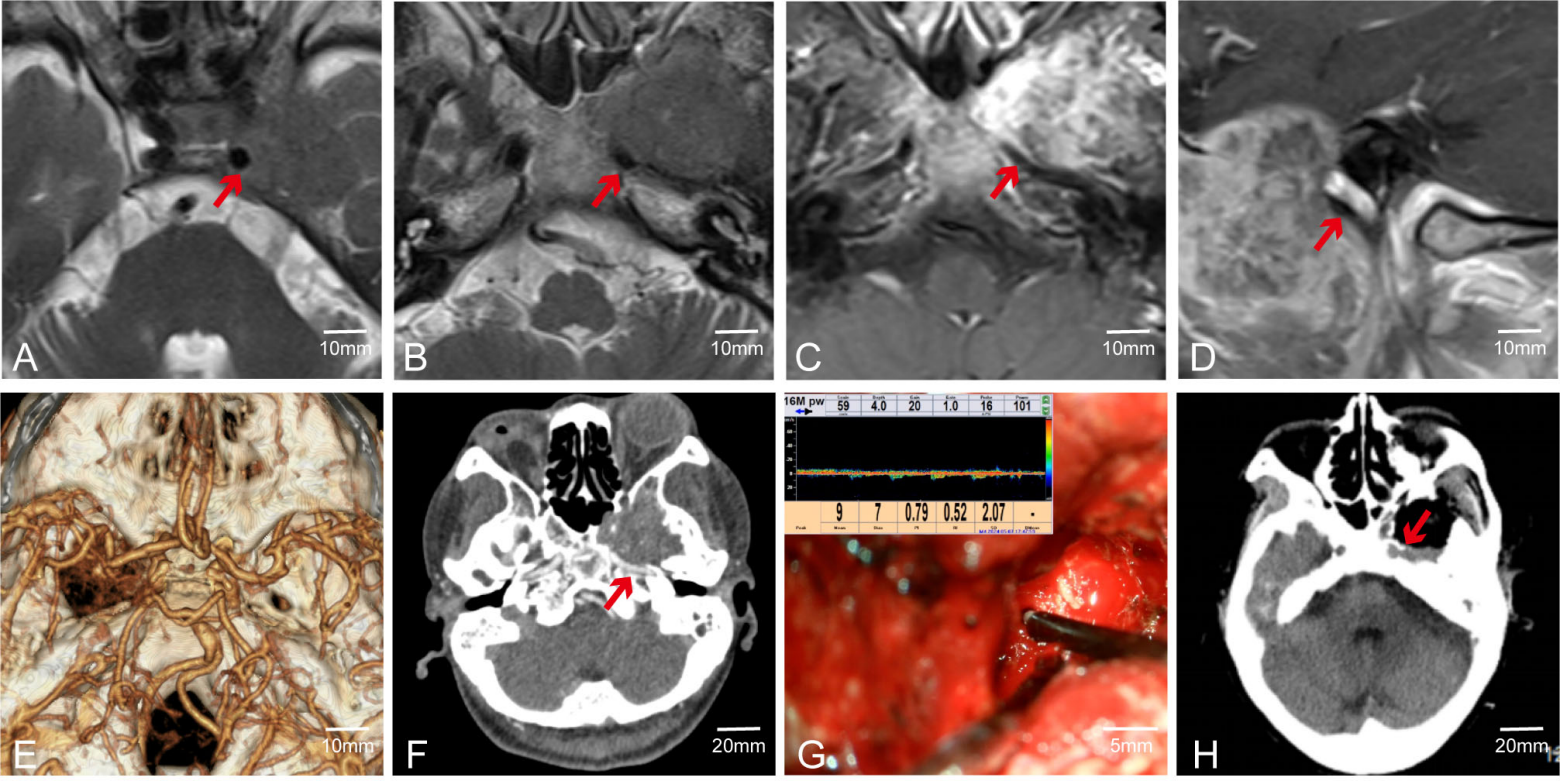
Case 55. MVD combined with CTA was used to evaluate the vascular condition of patients. (A–D) Preoperative MR showed that the ICA was closely related to the tumor. (E, F) Preoperative CTA. (G) Intraoperative fail to detect internal carotid artery. (H) Postoperative CT showed ICA lateral has a thin layer of bone.
